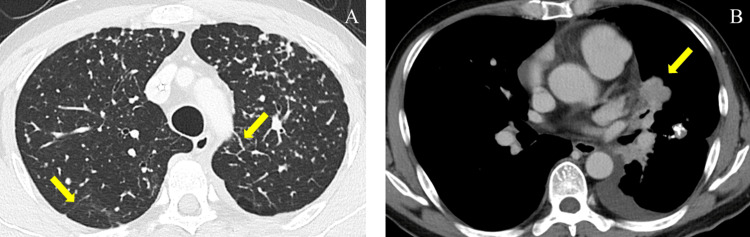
Chest computed tomography on admission. (A) Chest computed tomography imaging revealed military metastases and interlobular septal thickening in both lung fields (yellow arrows). (B) Minor pleural effusion and slight tumor progression (yellow arrow).
